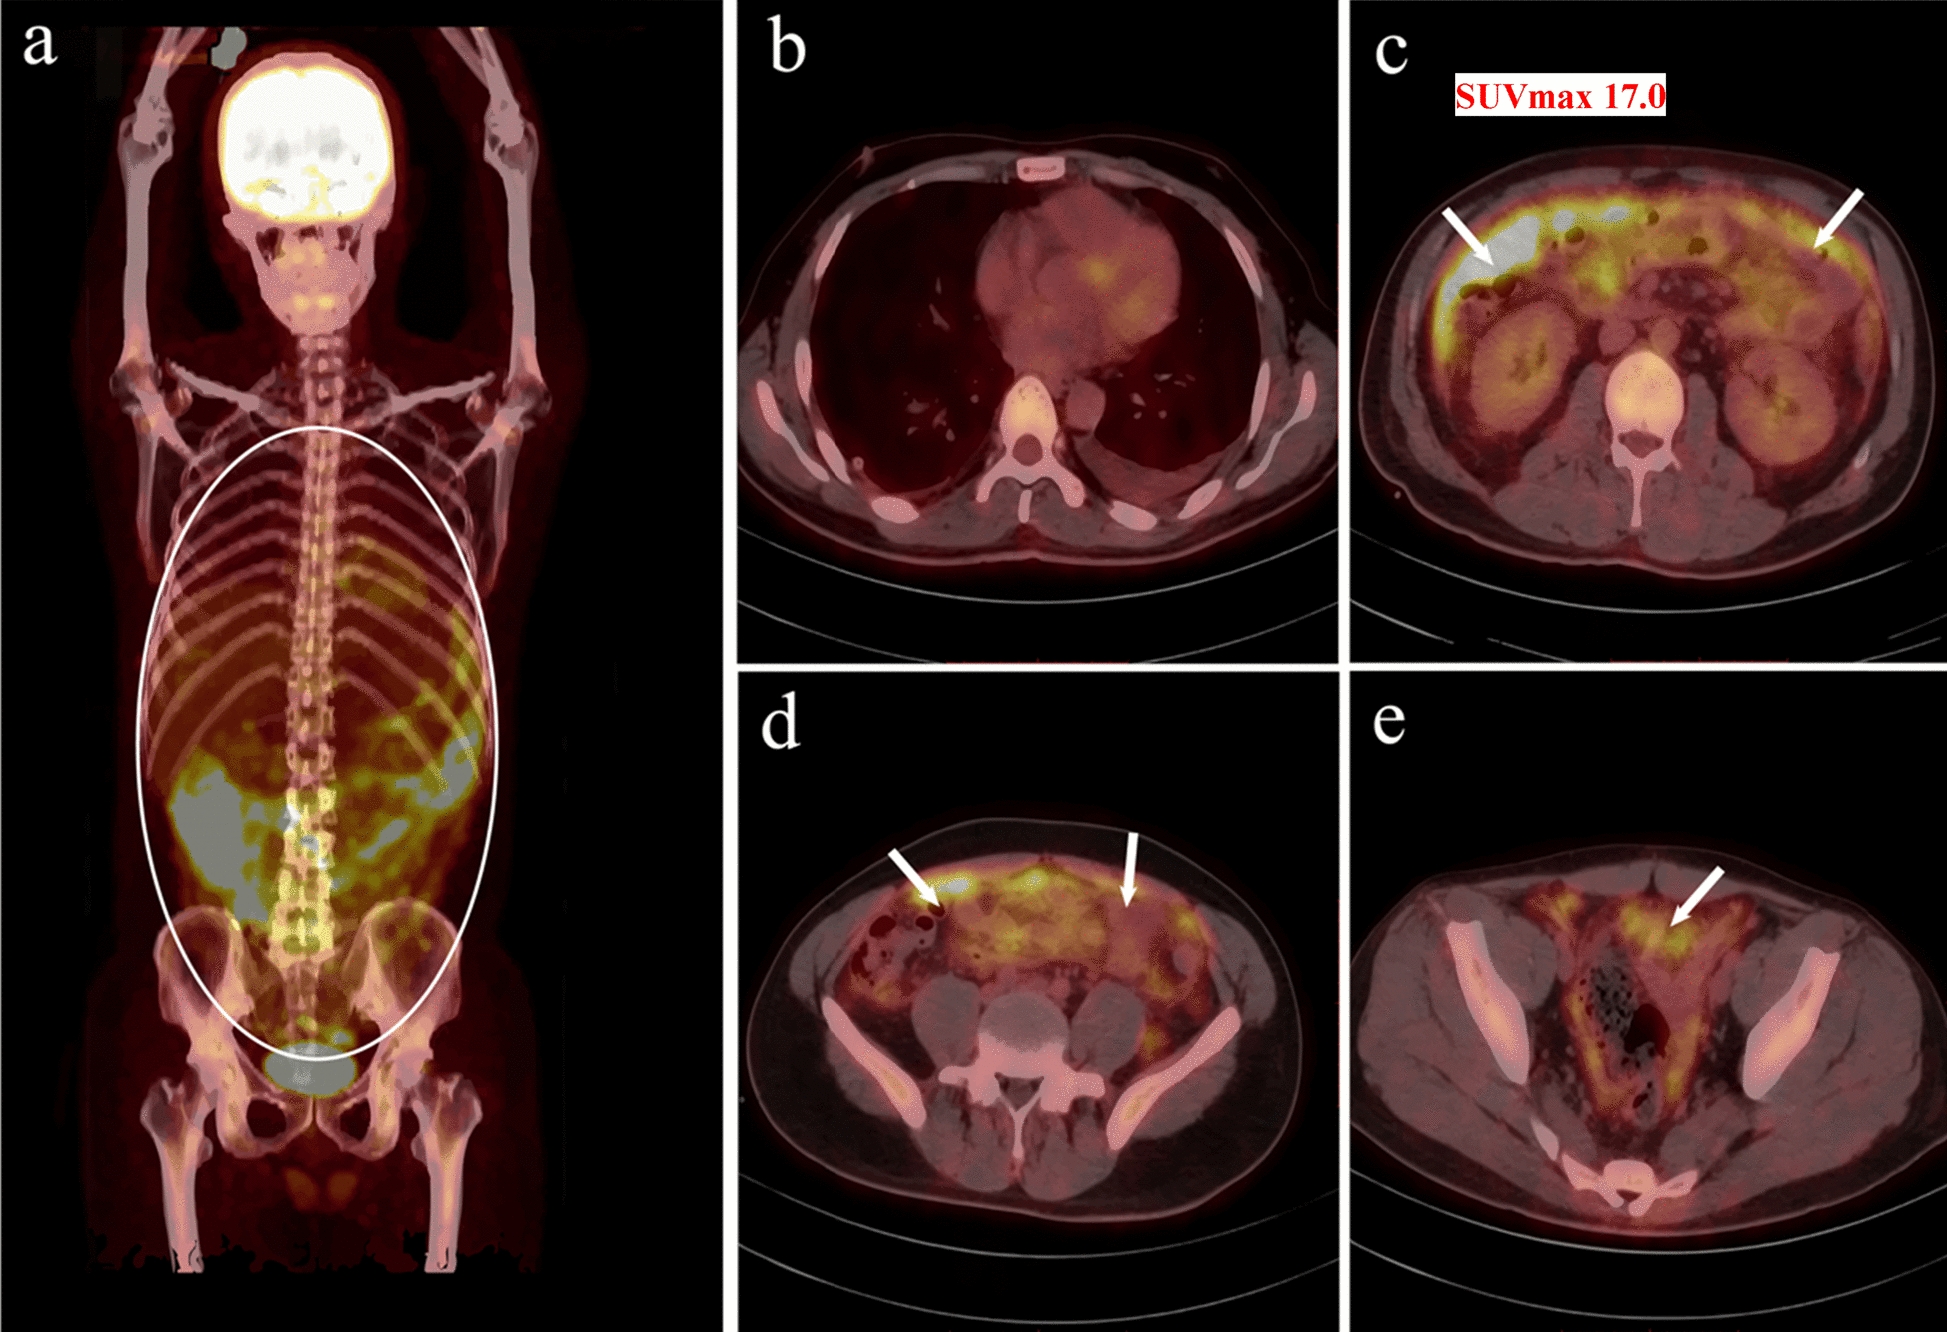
18F-fluorodeoxyglucose positron emission tomography (18F-FDG PET) demonstrating differential tracer uptake across multiple lesions. a Yellow-highlighted area reveals intense focal 18F-FDG uptake in local tissue (lesion marked with a circle). b No abnormal FDG metabolic activity is observed in the chest. c, d, e Marked hypermetabolic foci are noted in the peritoneal region (predominantly involving the greater omentum) and pelvis, with a maximum standardized uptake value (SUVmax) of 17.0 (indicated by arrows)
